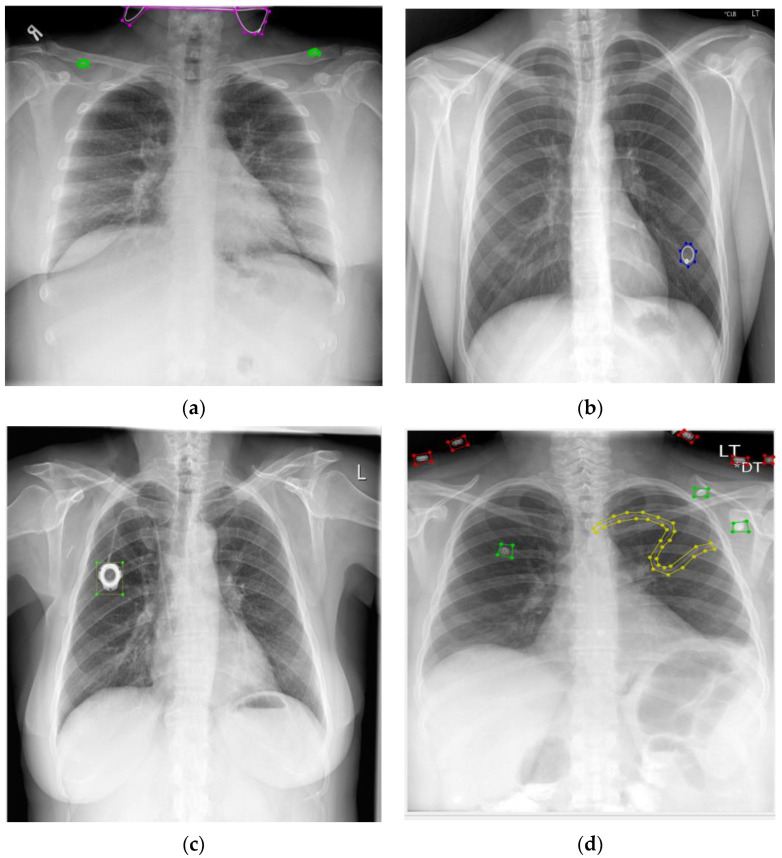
Foreign objects (annotated/labeled) in chest X-rays: (a) jewelry and buttons, (b) ring, (c) pacemaker, and (d) medical tube.
